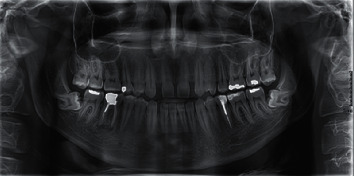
A panoramic radiograph of a patient with bilateral CMMLI.
